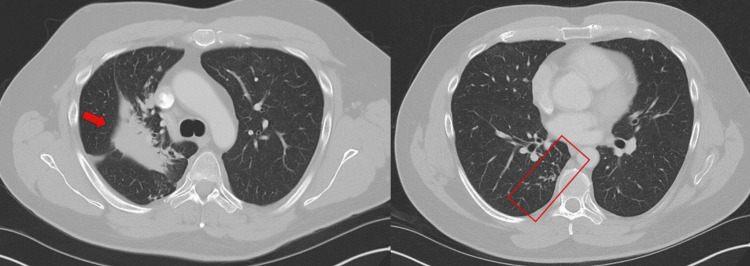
Noncontrast CT of the chest demonstrating residual fibroatelectasis (arrow) and tree-in-bud opacities (box).
